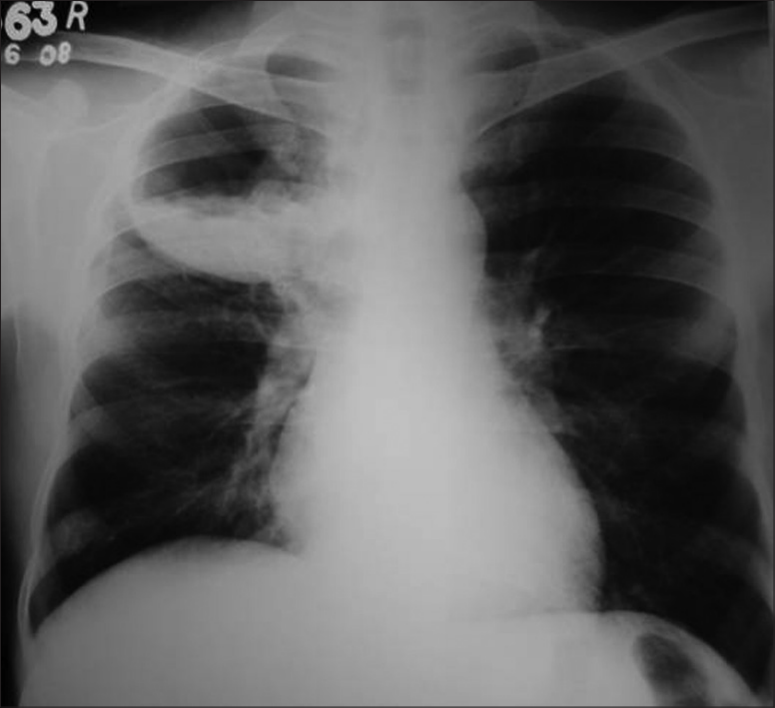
X-ray chest PA showing cavity with fluid level
